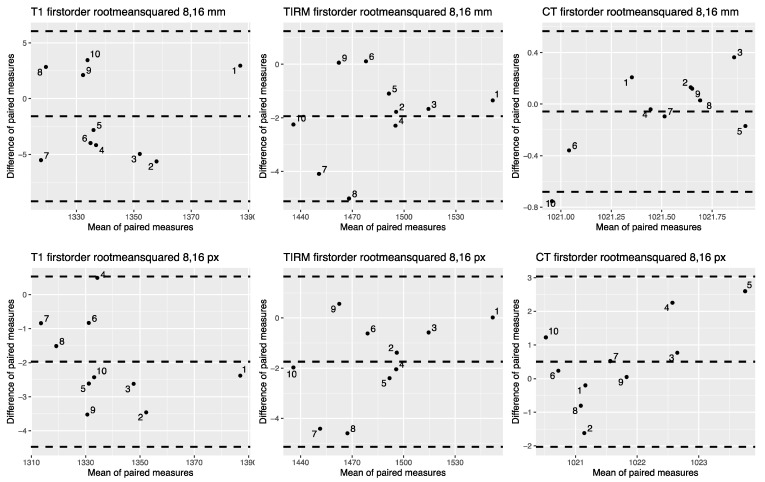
Bland–Altman plots for the first-order feature root mean squared for pixel-sized and mm-sized ROIs from CT and MR images. Bland–Altman plots for T1w MR images on the left, for T2w TIRM images in the middle, and for CT-derived images on the right side. The upper row shows root mean squared extracted from mm sized ROIs, the lower row root mean squared extracted from px sized ROIs. Bland–Altman plots for all features are shown in the Supplementary Materials (see Figure S5).
